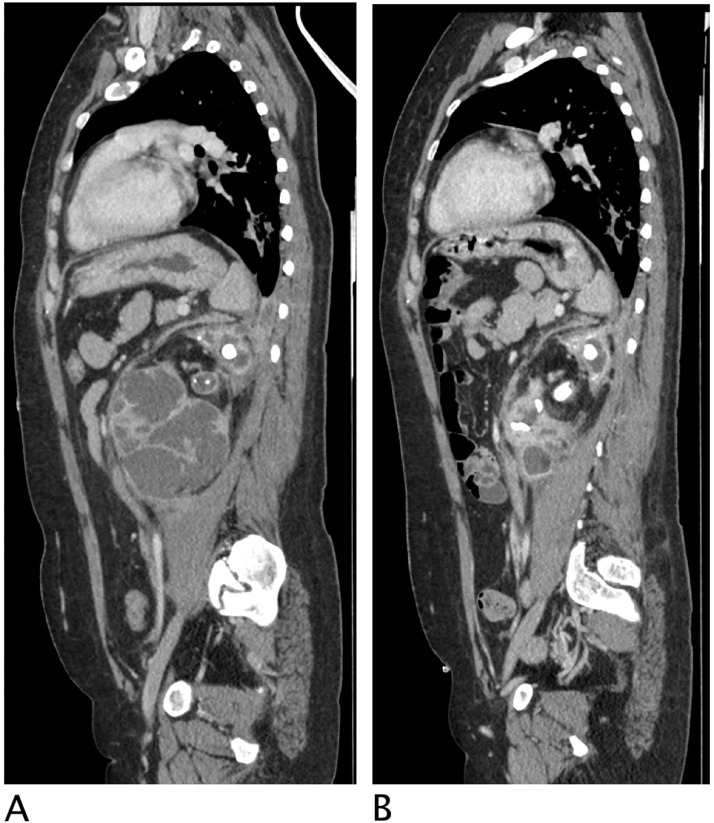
CT with intravenous contrast, parenchymal phase. Multiplanar sagittal plane reconstruction . (A) Admission CT Left markedly dilation of calico- pelvic system with calyces corticalization. , A fistulous connection is clearly visible between the upper pole of the left kidney through the diaphragm. (B) CT after 15 days of antibiotics therapy and stent placement. The fistulous connection is no more appreciable, a soft tissue band with moderate enhancement is visible. The calico pelvic system appears significantly decompressed.
